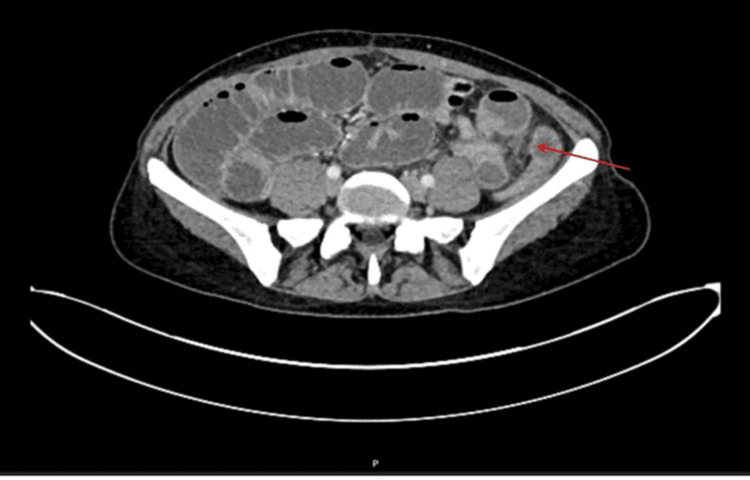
Computed tomography of the abdomen and pelvis showing dilated small bowel loops with transition point at distal ileus loops in the left iliac fossa (red arrow)
